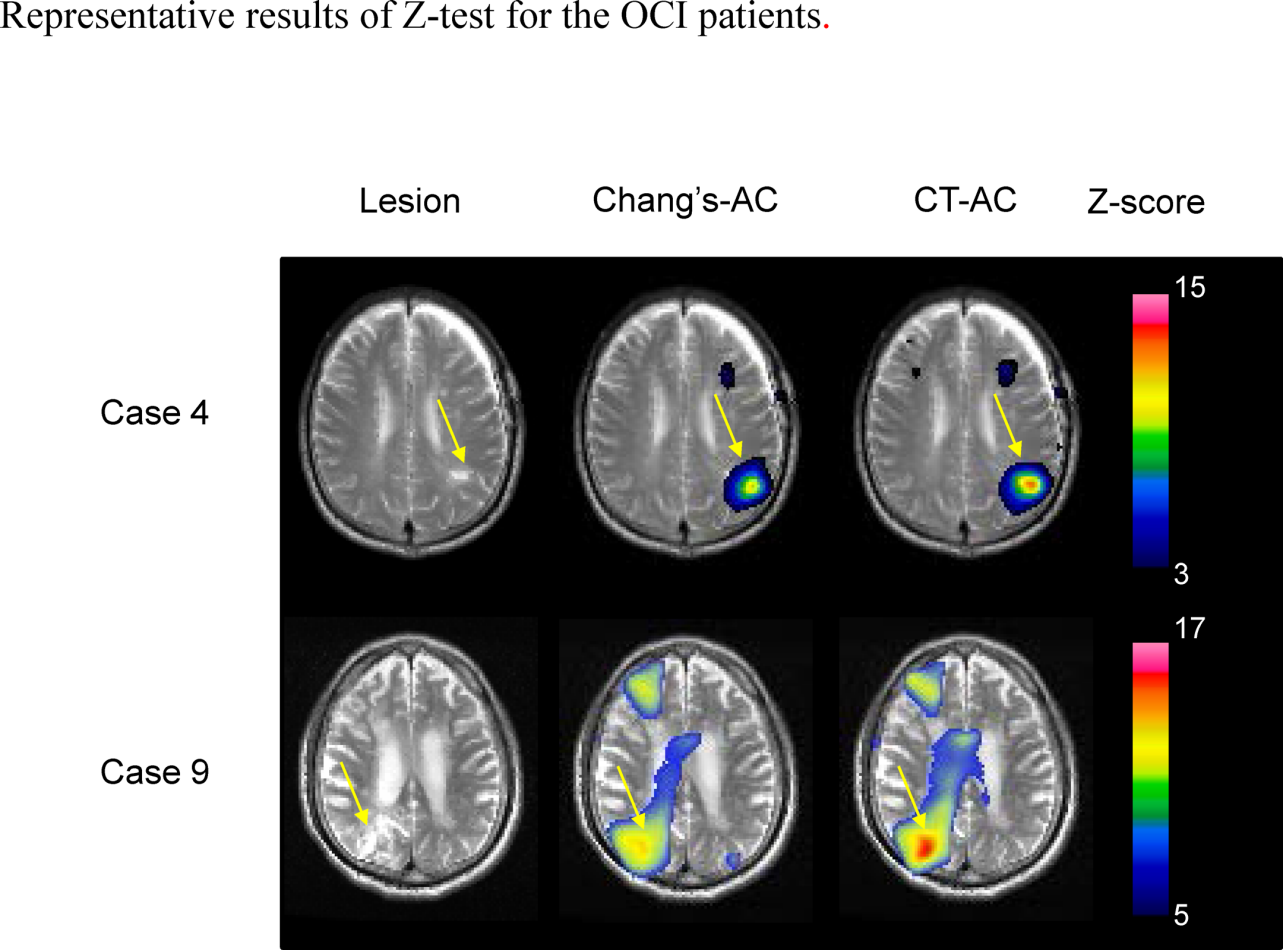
Results of Z-test for representative cases of OCI patients: (A) case 6 and (B) case 9. Lesions of each case are shown by MRI in the leftmost column. The Z-score maps by Chang’s-AC and CT-AC overlaid on the normalized MRI of each patient are displayed in the center and the rightmost column respectively.
